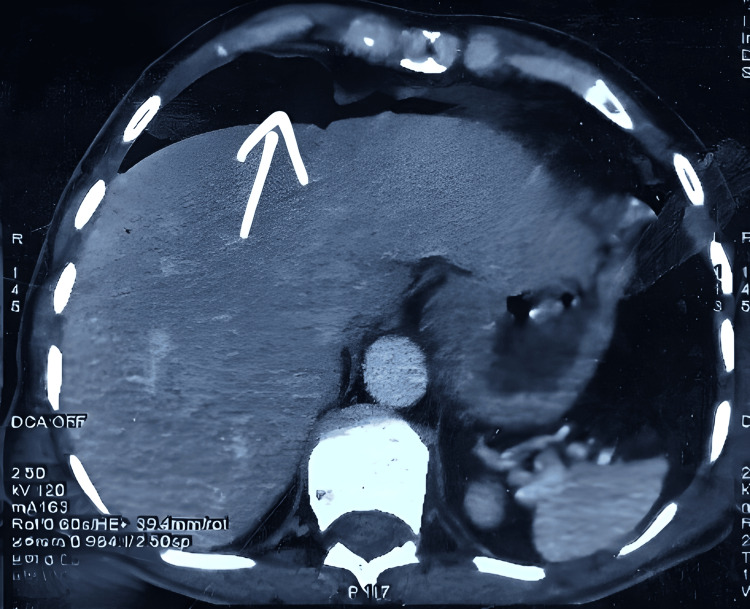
Axial CT scan showing pneumoperitoneum. Significant pneumoperitoneum with free air (white arrow) indicates bowel perforation, appearing as hypodense areas throughout the peritoneal cavity.
